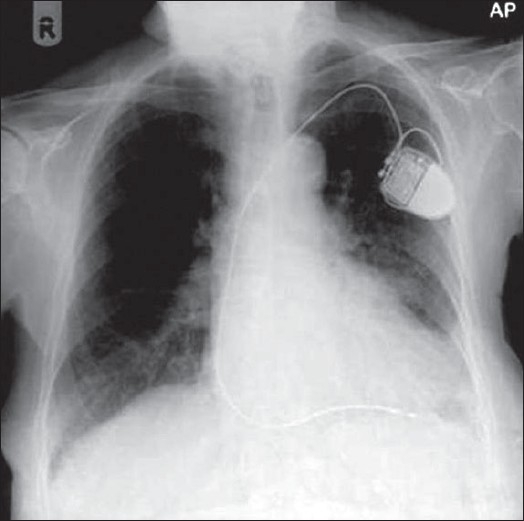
A check radiograph following placement of a cardiac pacemaker shows the position of electrode to lie within the apex of the right ventricle
